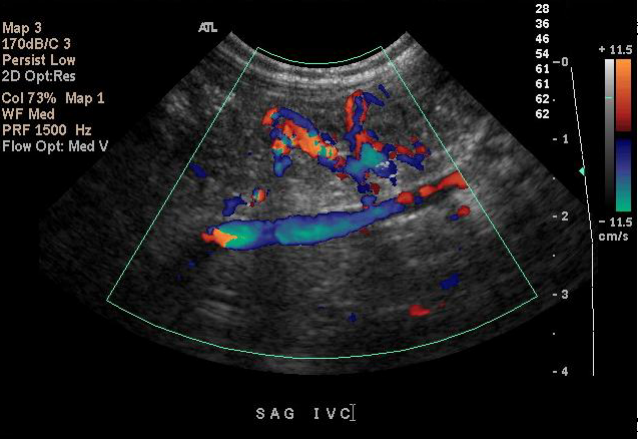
Follow-up renal Doppler ultrasound flow at 2 months of age showing the normal flow pattern of the inferior vena cava.
